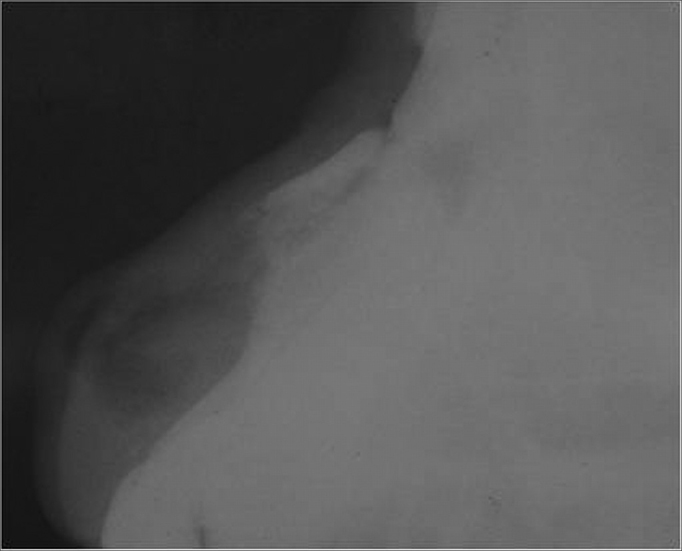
Lateral nose radiograph - immediate postoperative.
